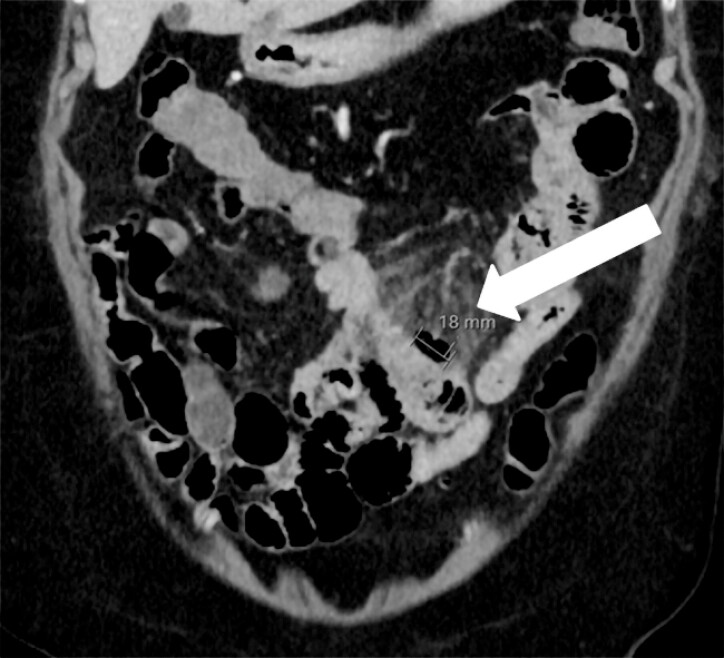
Abdominal computed tomography (post-perforation) showing fat-stranding and an 18 mm diverticulum.
